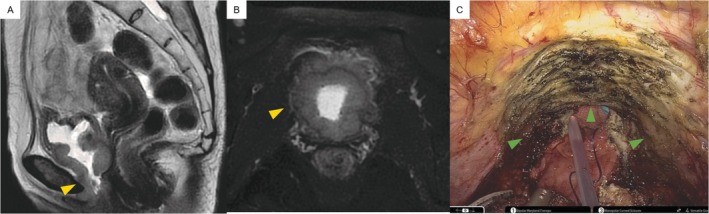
Sagittal (A) and axial (B) T2‐weighted magnetic resonance images of Case 3 before chemotherapy. The yellow arrows indicate a mass extending from the bladder neck to the external urethral meatus, with irregular wall thickening and urethral dilatation. The tumor was in close proximity to the levator ani muscle, pubic bone, and vaginal wall, suggesting invasion. (C) The green arrows indicate that the pubic periosteum and the levator ani muscle were resected en bloc.
